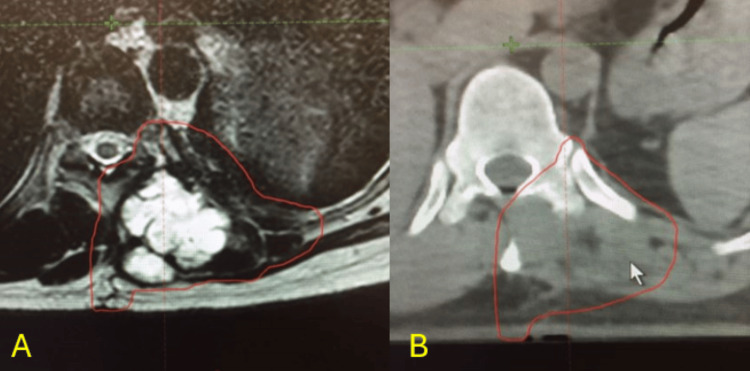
Pre-operative planning to mark the boundaries of excision Radiographic imaging used during surgical planning to mark the boundaries to perform the en-bloc excision of the tumour 7A: Tumour delineation under MRI 7B: Tumour delineation under CT scan
